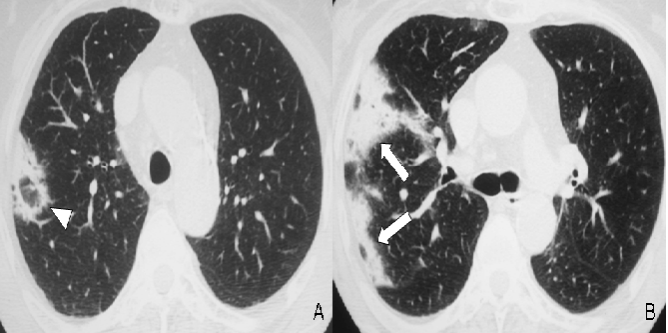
High-resolution computed tomography (HRCT) two months after discontinuation of treatment at the level of the aortic arch (A) and the carina (B) at lung windowing shows deterioration of the disease with extensive subpleural and peribronchovascular consolidation in the right upper lobe (arrows) and with new ring-like opacities consistent with the "reversed halo sign" (arrowhead).
